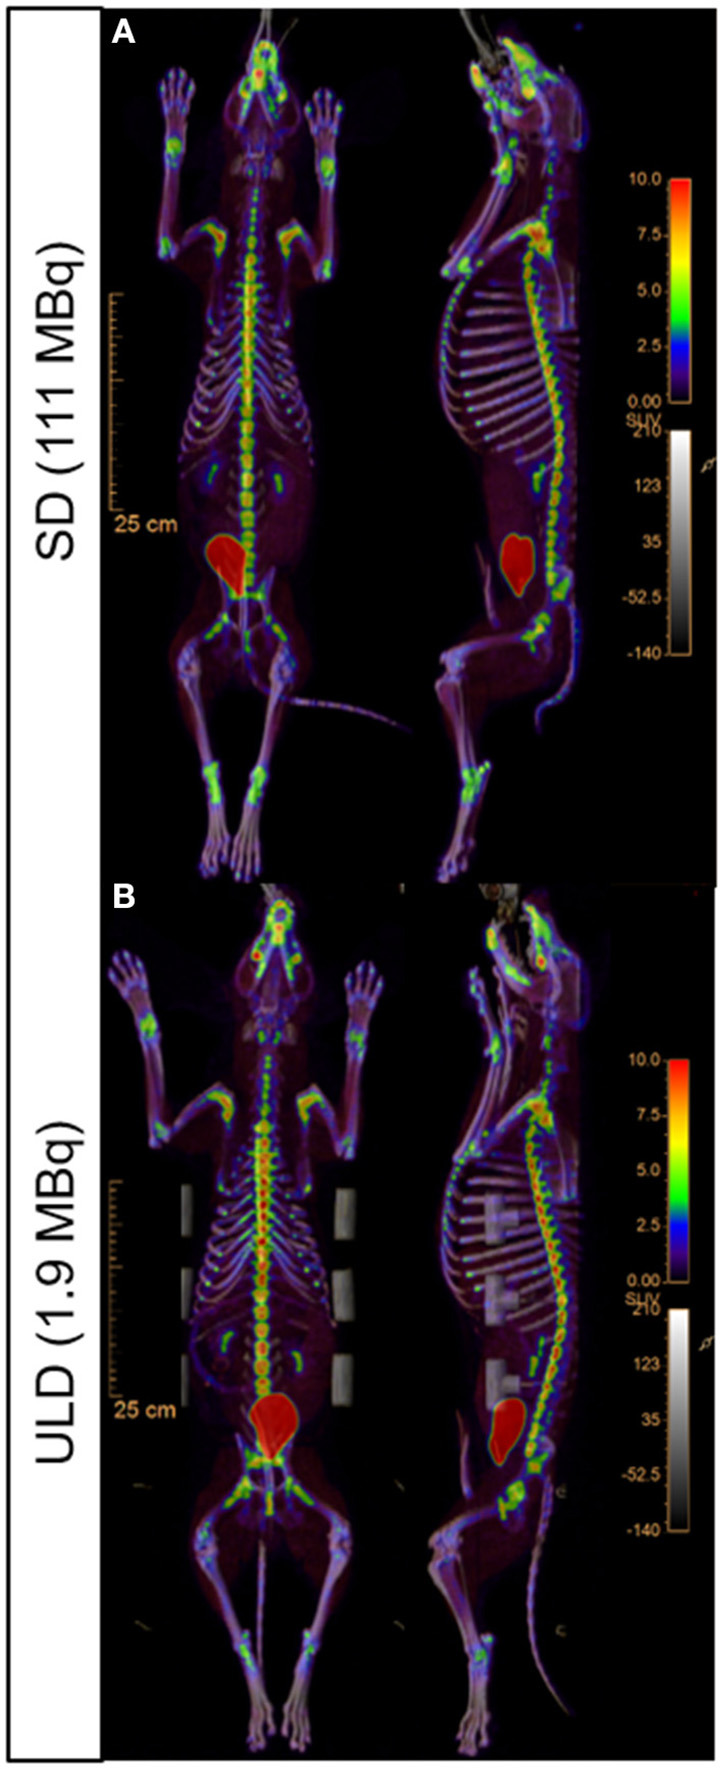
Representative whole-body [^18^F]NaF PET/CT uptake fusion showing the metabolic bone activity. Standard dose [SD; (A)] and ultra-low dose [ULD; (B)] dorsal (left) and sagittal (right) images.
